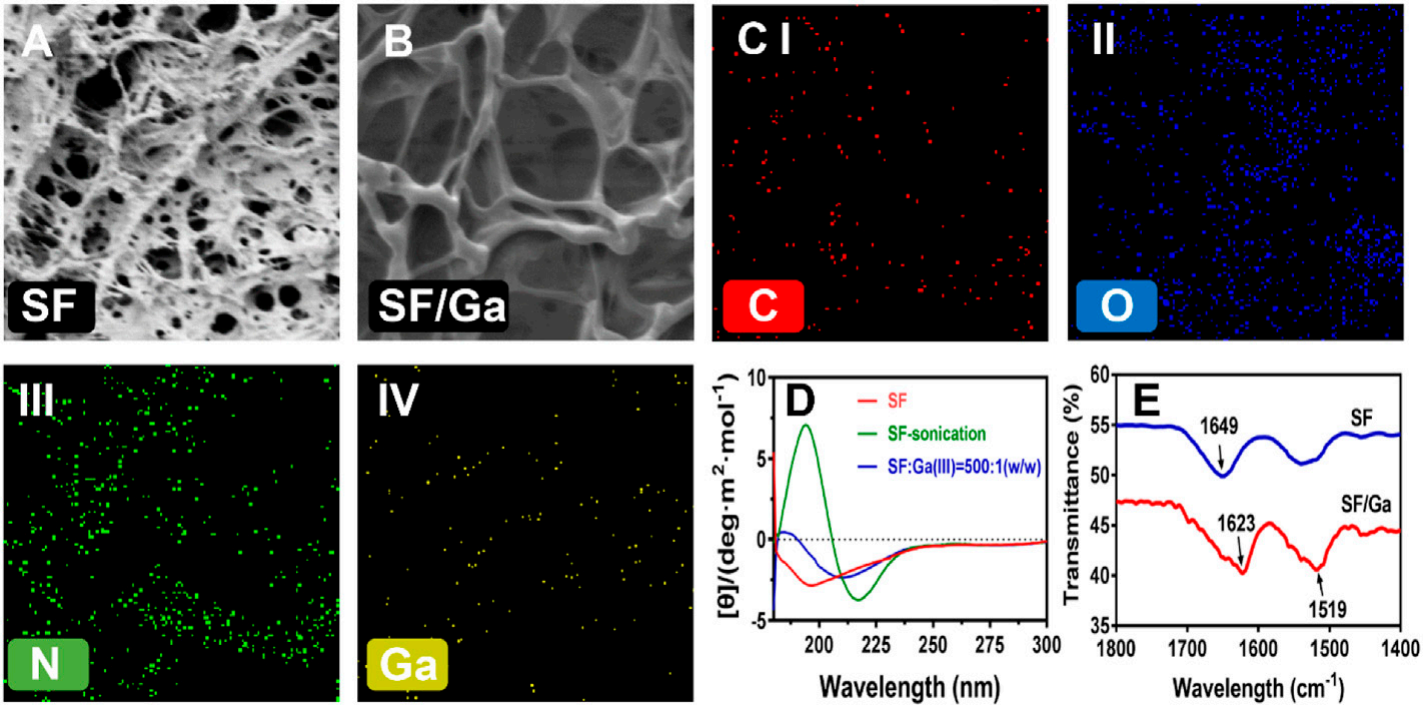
Morphological and secondary structural characterization. (A,B) SEM images of the surface pore of SF and SF/Ga hydrogel, (C) Elemental mapping of SF/Ga hydrogel, (D) CD spectrum of unsonicated SF, ultrasonicated SF, and sonicated SF/Ga solutions, (E) FTIR of SF and SF/Ga hydrogel.
